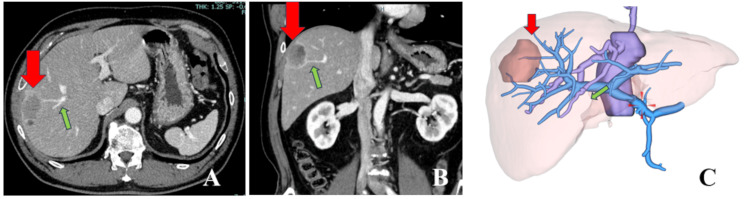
Preoperative images A and B: Findings of preoperative contrast-enhanced computed tomography. C: 3D construction of the liver (red arrow heads indicate the tumor; green arrows indicate the G8 branches). The tumor measured 42 mm and was located in segment 8 of the liver. It extended deep within the liver, and at a distance of 1 cm from the deepest part, a branch of the portal vein (G8) was present. The plan was to perform a liver resection targeting this area.
